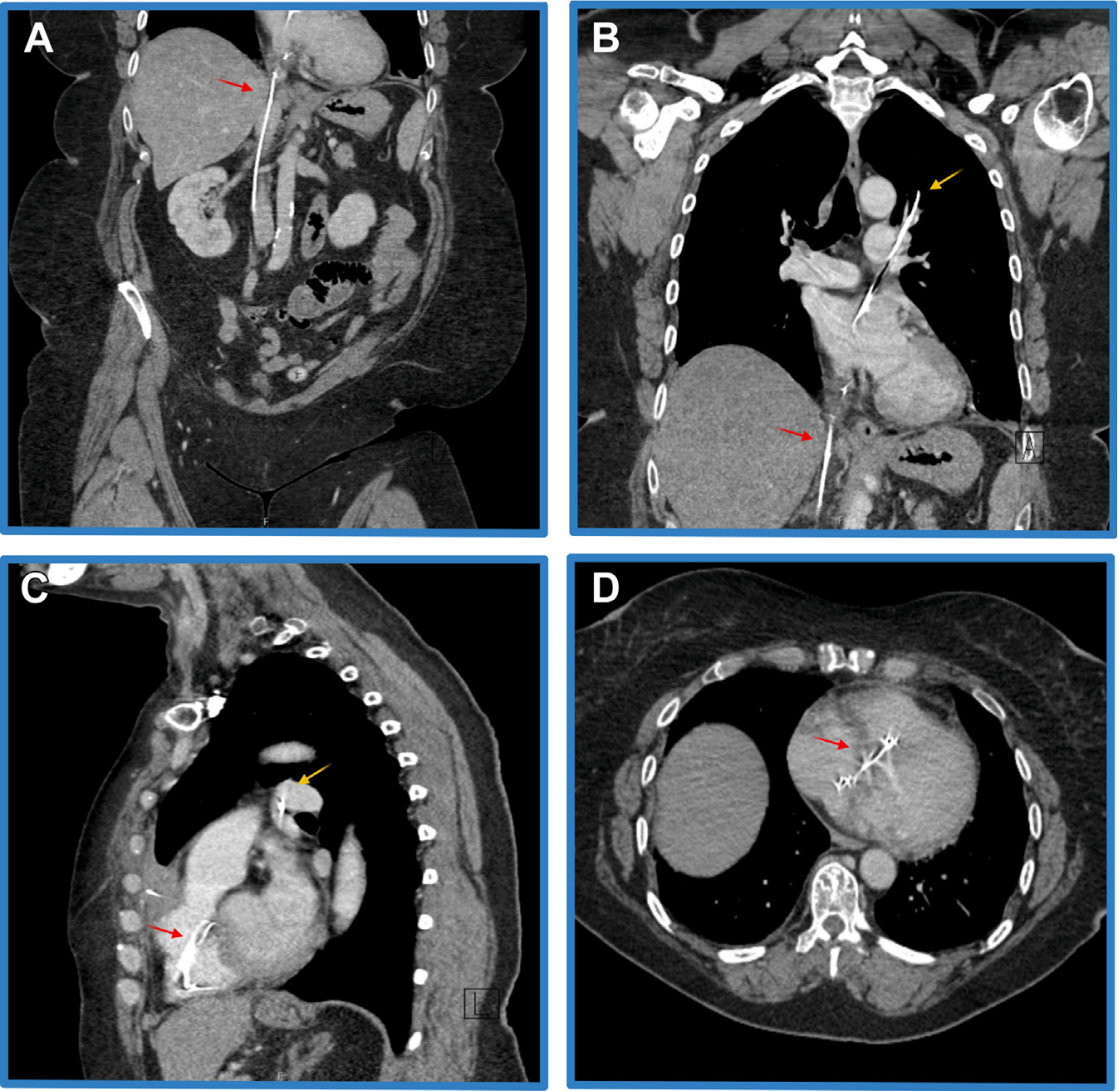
Intravascular Course of the Wire, With 4 Separate Still Images Taken From Computed Tomography of Different Slices Transverse thorax slice showing the wire crossing the atrial septum (red arrow). (A) Coronal abdominal slice. Red arrow indicates a wire within the inferior vena cava extending up into the right atrium. (B) Coronal thorax slice. Red arrow shows the wire entering the heart via the inferior vena cava, traversing the septum, and reaching the upper left pulmonary vein (yellow arrow). (C) Sagittal thorax slice. Red arrow highlights filaments of the unraveled wire within the right ventricle. Yellow arrow indicates the wire within the pulmonary venous vasculature, again confirming it has crossed the septum. (D) Transverse thorax slice. This view clearly shows the wire crossing the atrial septum.
